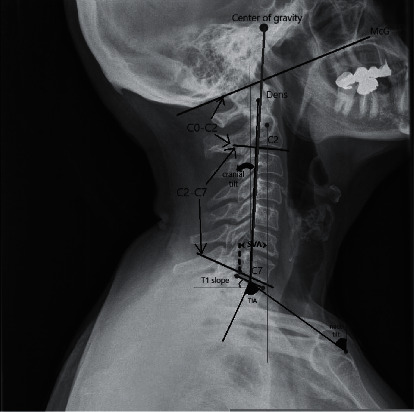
Lateral roentgenographic parameters used for sagittal cervical balance study.
